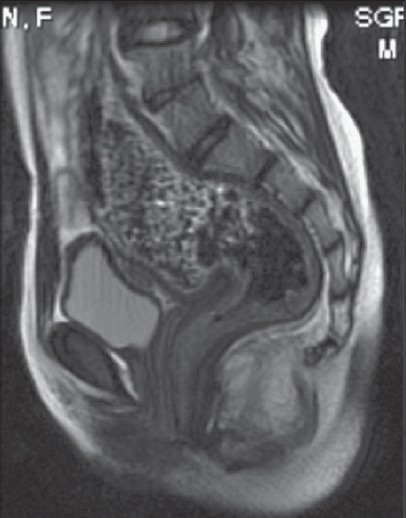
MRI picture of an 11-year-old girl with anovestibular fistula
